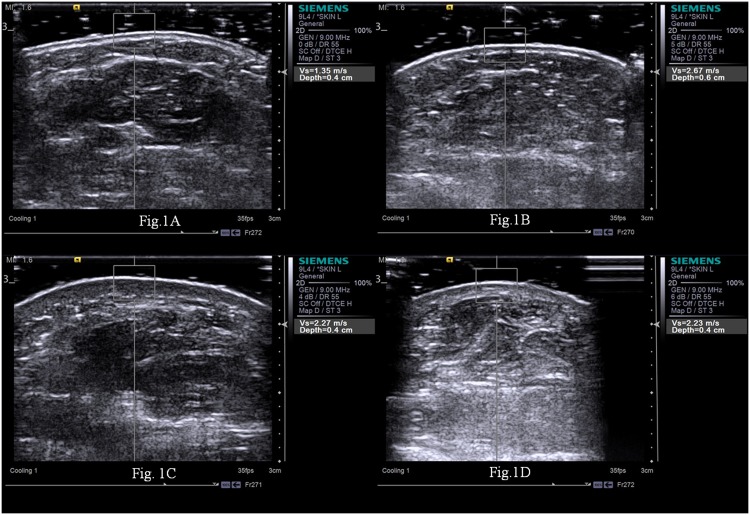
Ultrasound findings of the dcSSc patients and the control’s right forearm skin. The echogenicity was determined as compared with site-matched, normal skin in healthy controls (Fig 1A), and was classified into isoechogenic (Fig 1B), hypoechogenic (Fig 1C), and hyperechogenic (Fig 1D).
